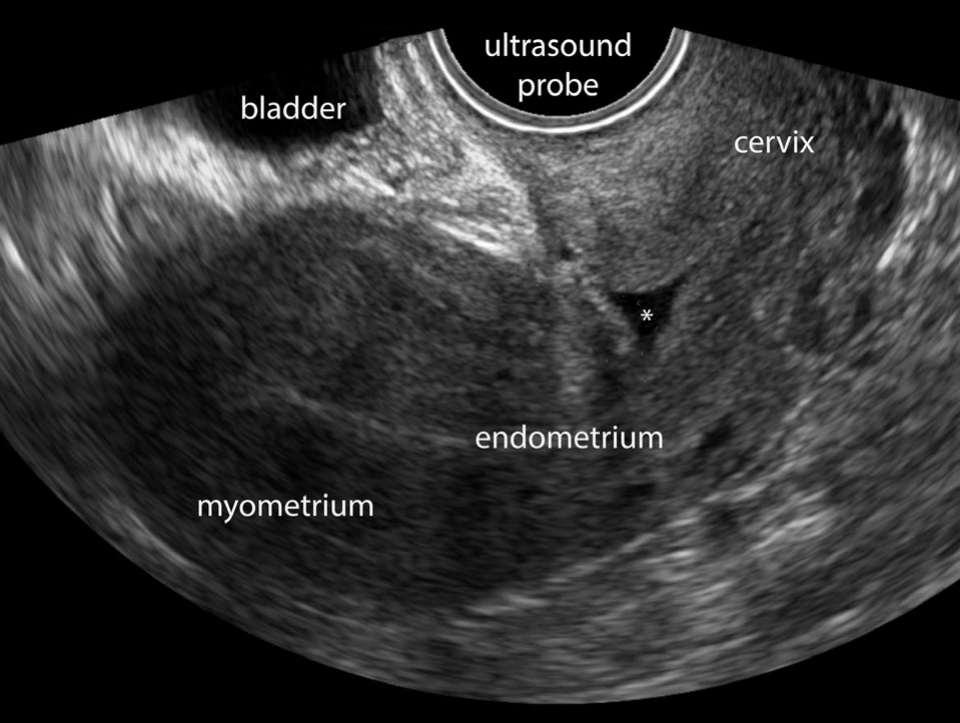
Niche after caesarean section on transvaginal ultrasound, without the use of contrast. A niche is visible, indicated with an asterisk *, in the caesarean uterine scar.
